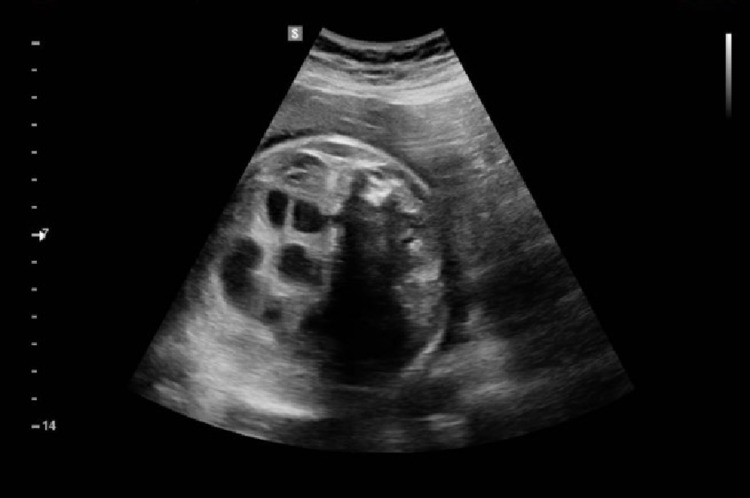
Antenatal ultrasound at 31/40 gestation showing normal calibre of distal bowel with dilated proximal bowel loops
